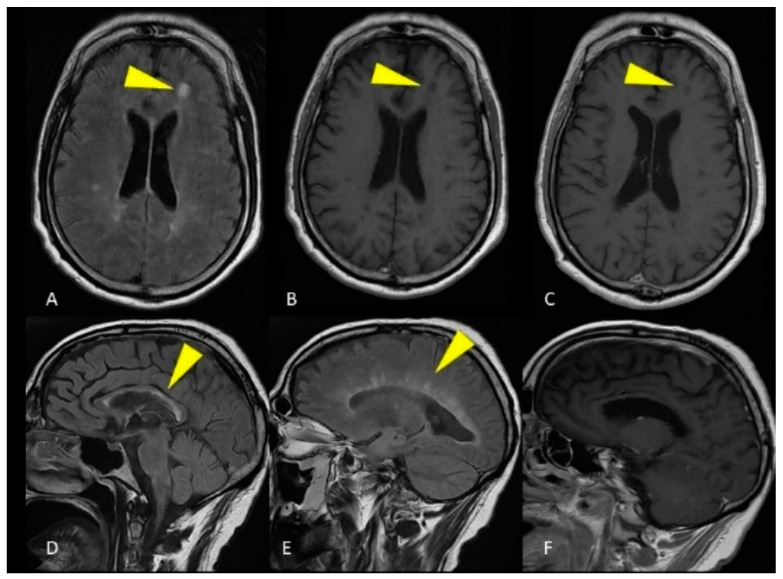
MRI of the brain. Axial FLAIR image (A) and unenhanced T1-weighted image (B) show a single ovoid-shaped lesion in the left frontal deep white matter, without enhancement on the T1-weighted post-gadolinium acquisition (C). Sagittal FLAIR image shows hyperintense lesions affecting the ependymal surface of the corpus callosum with a marble pattern (D). The so-called Dawson fingers are visible on the sagittal FLAIR image as hyperintense, ovoid lesions perpendicular to the body of the lateral ventricle (E). Corpus callosum and periventricular lesions don’t show enhancement on the T1-weighted post-gadolinium acquisition (F).
